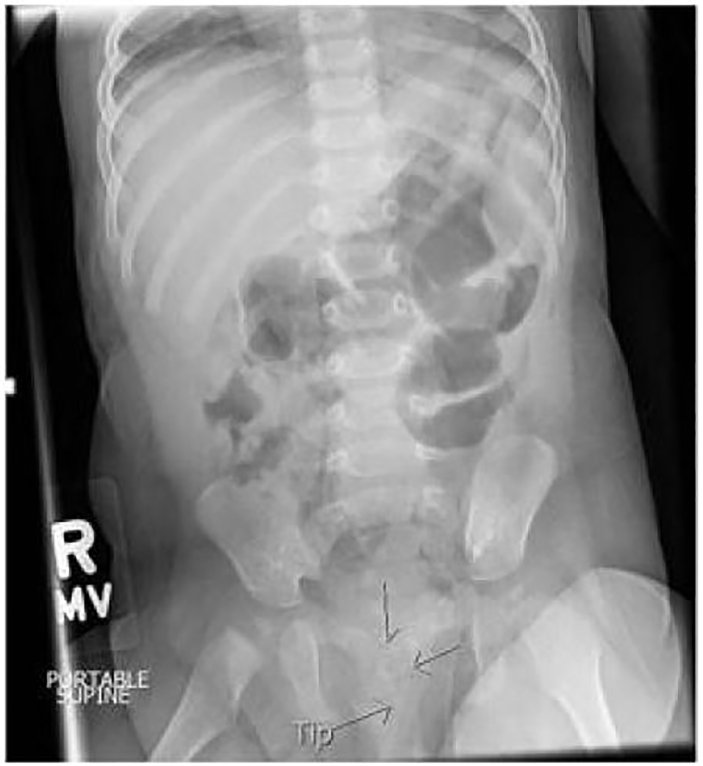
Anteroposterior abdominal radiograph (arrows delineate the catheter location with knot)
